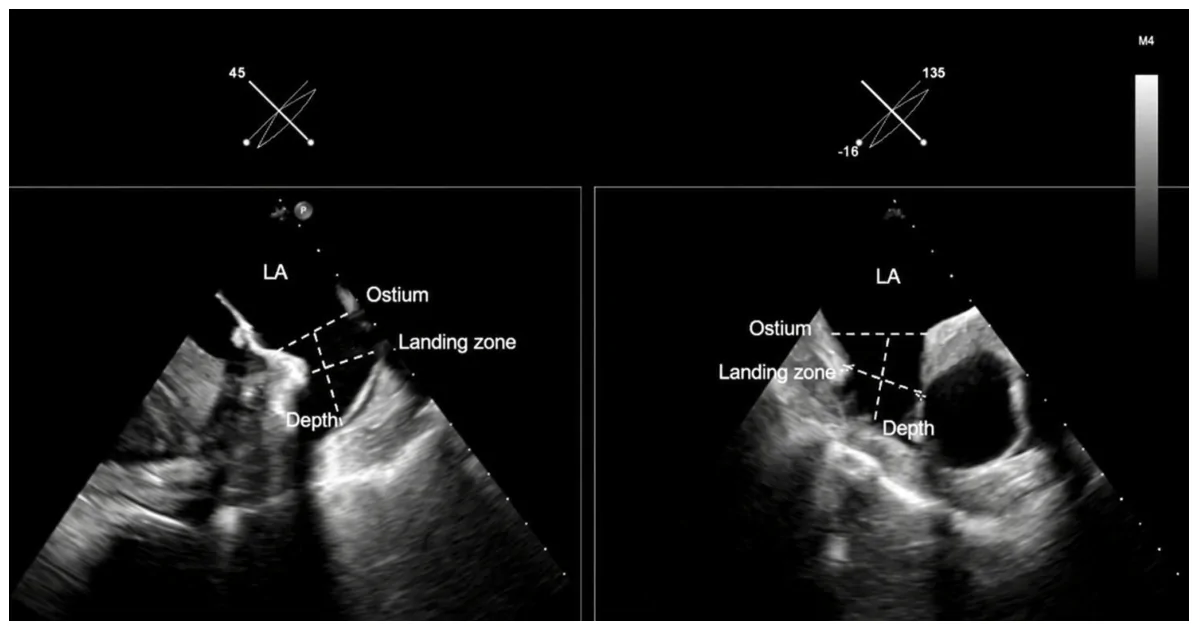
LAA sizing using 3D TEE. The LAA orifice diameter and depth are measured at 0°, 45°, 90°, and 135°. The LAA landing zone is measured from the top of the mitral valve annulus or circumflex coronary artery to a point 2 cm below the tip of the left upper pulmonary vein limbus, while depth is measured from the plane of the LAA orifice to the LAA apex. 3D, three-dimensional; LAA, left atrial appendage; TEE, transesophageal echocardiography.
